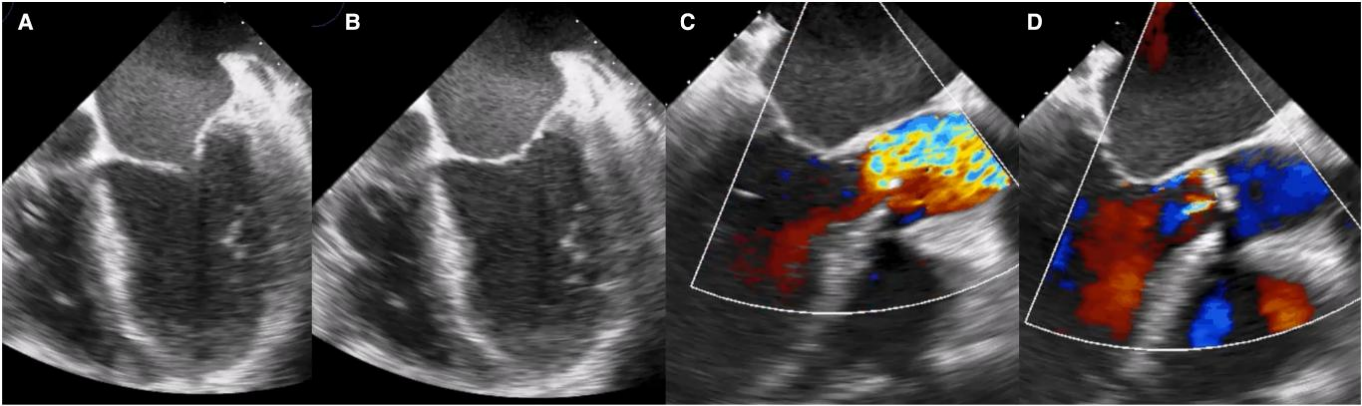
Transoesophageal echocardiogram showing the left ventricle with decreased generalized mobility, (*A*) ventricular systole, (*B*) ventricular diastole, (*C*) turbulent flow secondary to valve obstruction is observed, and (*D*) mild aortic insufficiency.
